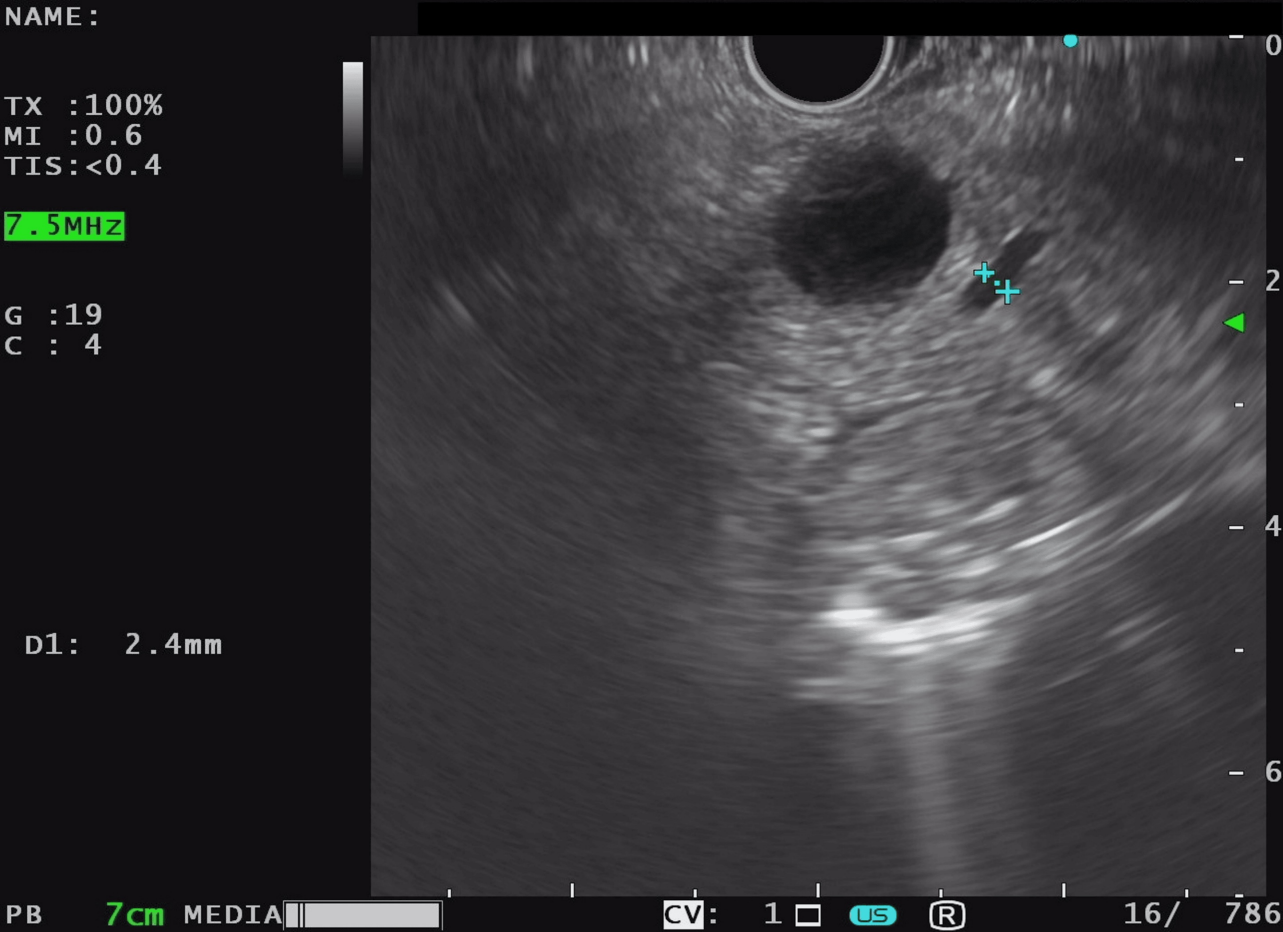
Endosonography The Wirsung duct is thin throughout its course, adjacent to the cystic lesion, which it surrounds, without any visible communication with the described lesion
